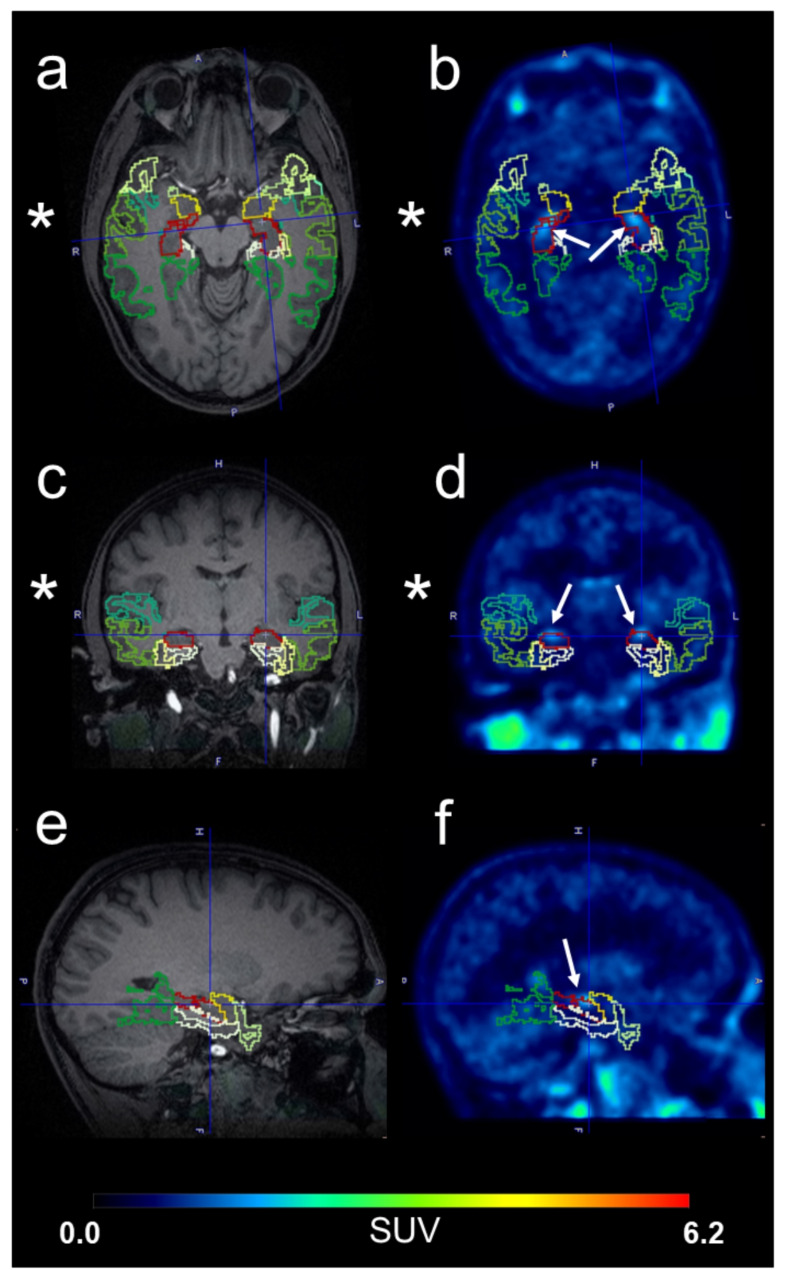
T1-weighted MR images (a, c, e) and averaged [^11^C]metoclopramide PET images (b, d, f) in 3 planes (from top to bottom: horizontal, coronal and sagittal [left hemisphere]) in one non-lesional drug-resistant epilepsy patient (p01-04) showing different temporal lobe sub-regions (green to yellow) from the brain region atlas (N30R83) and contamination of the hippocampus VOI (in red) by the choroid plexus (white arrows). The side of the seizure focus (right hemisphere) is indicated by an asterisk. Intensity scale for PET is expressed as standardised uptake value (SUV) and set from 0 to 6.2
